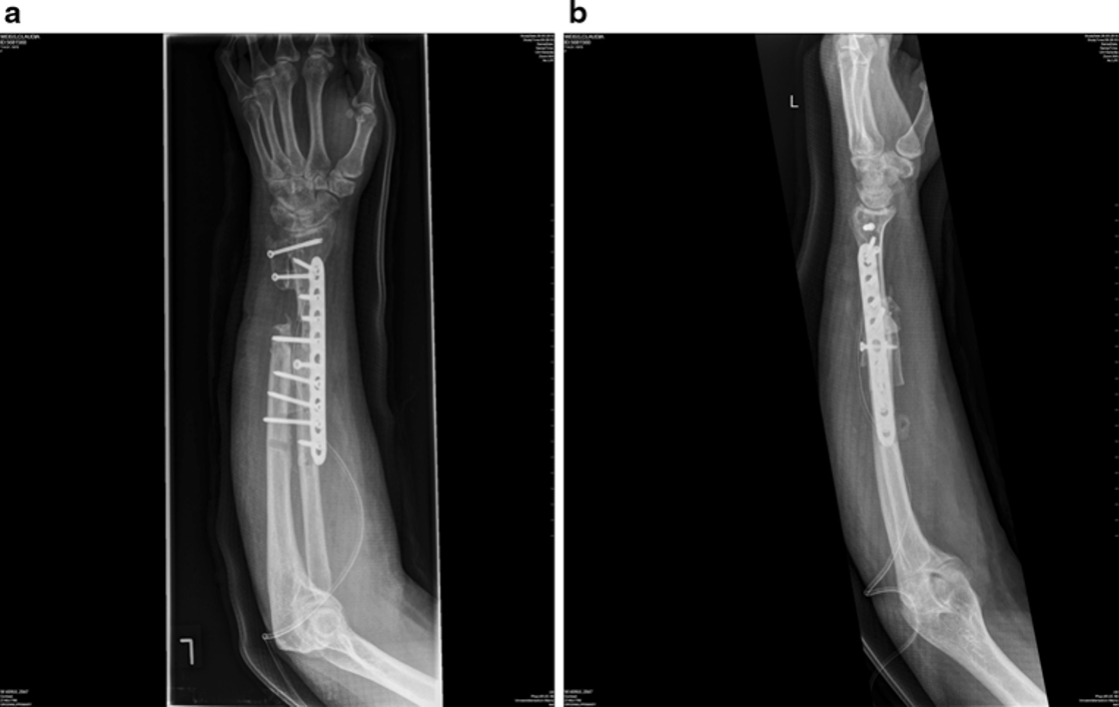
AP and lateral forearm radiographs after revision synostosis
